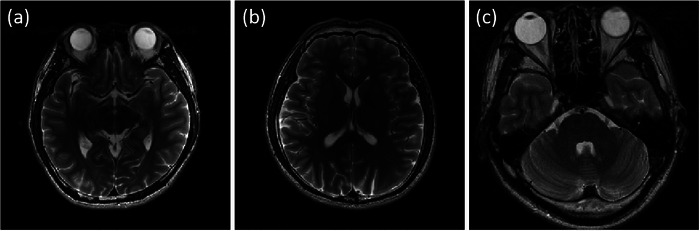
The experimental data of MRI. The parts of figure (a) and (b) are the 7th and the 27th slice of the brain MRI 1. The part (c) is the 31st slice of the brain MRI 2.
